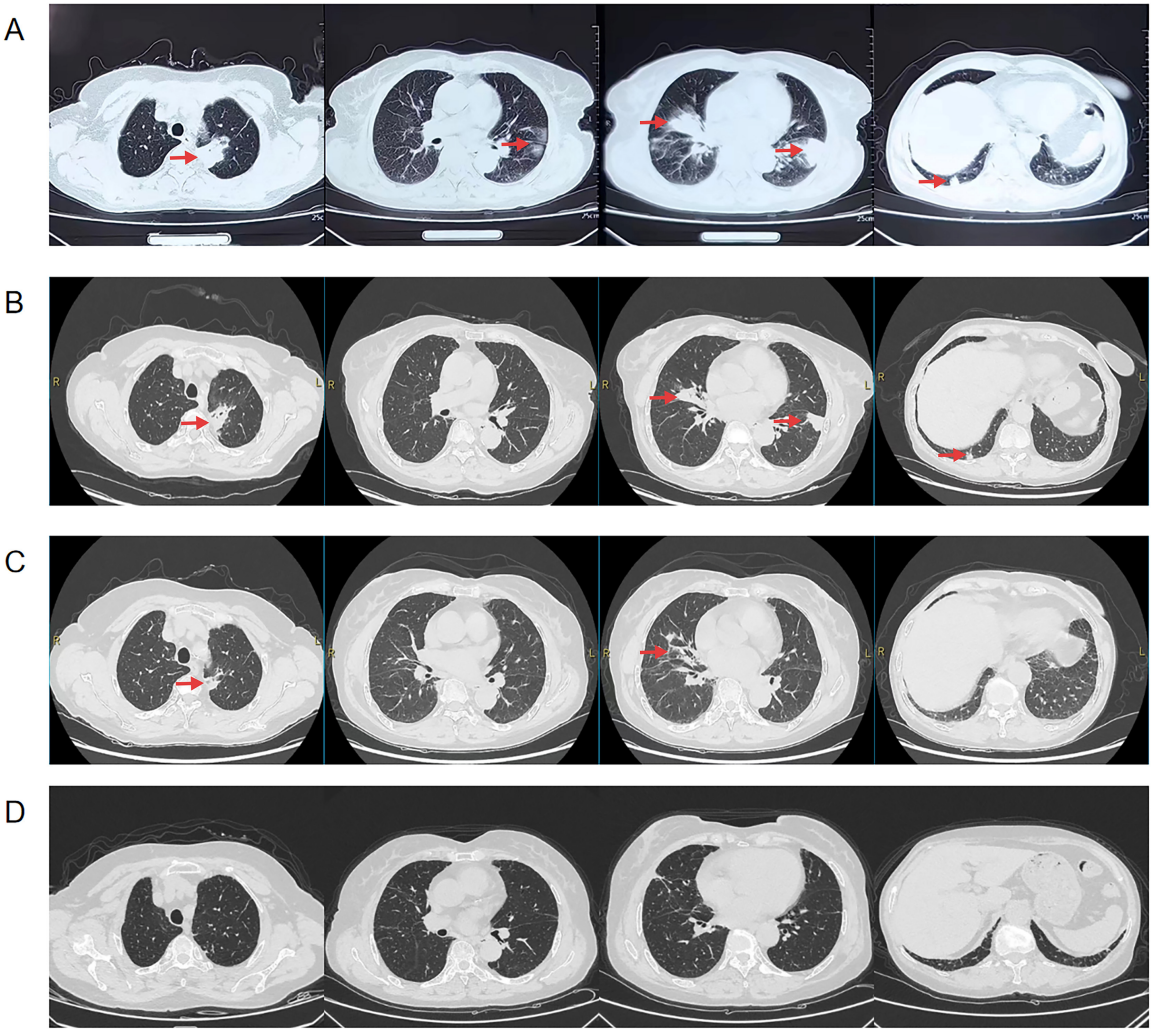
Chest CT of the patient before admission, 2 weeks of anti-infective treatment and after discharge, the location of the lesion is marked with a red arrow, (A) Pre-admission chest CT suggests multiple patchy, clumped, and ground-glass hyperdense shadows in both lungs, (B) Chest CT after 2 weeks of anti-infective treatment (amoxicillin/clavulanic) showed partial resorption of the lesion compared to the previous one, (C) Repeat chest CT 1 month after discharge suggests continued lesion resorption, (D) Chest CT 4 month after discharge suggests continued lesion resorption.
